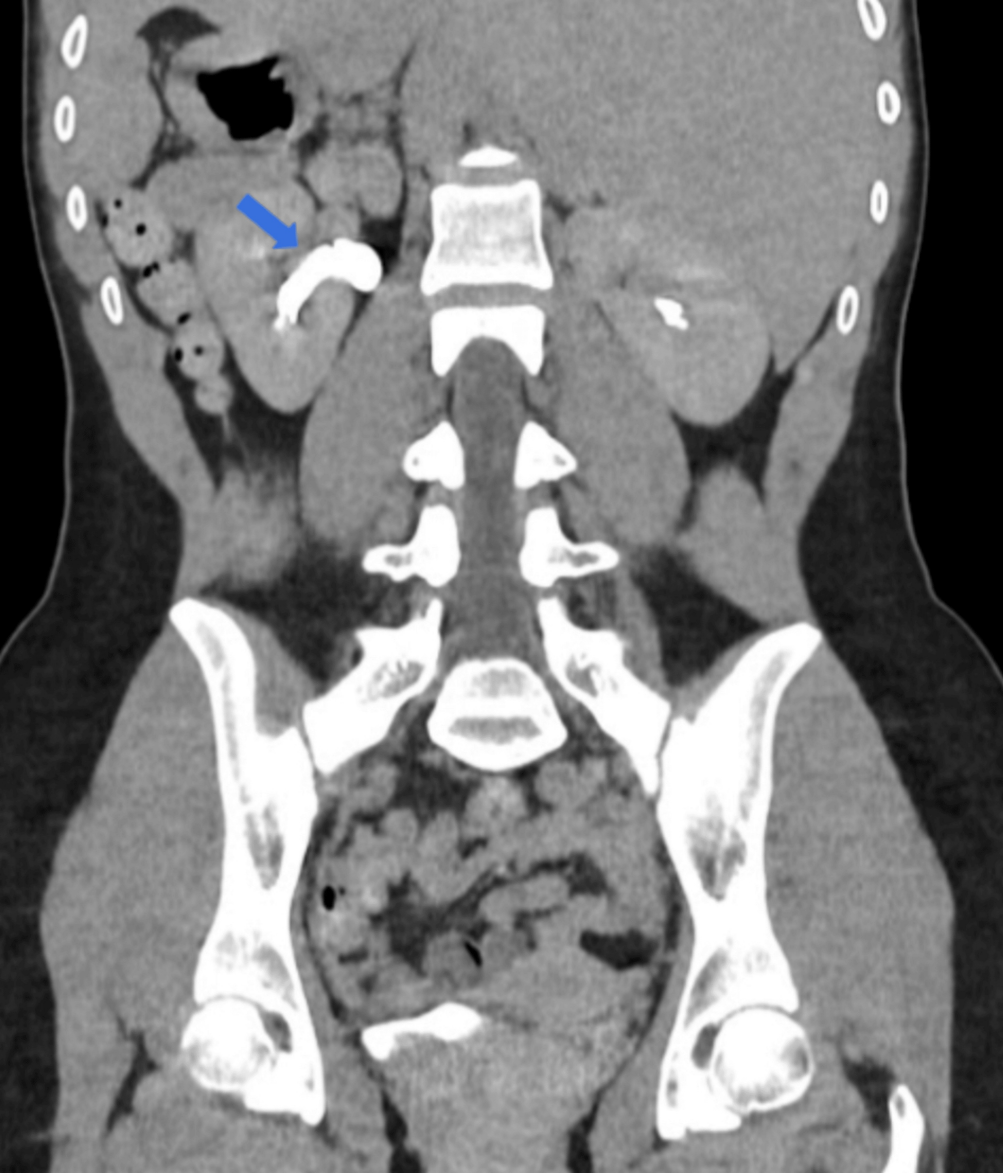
Left extrarenal pelvis (abdominal computed tomography)
